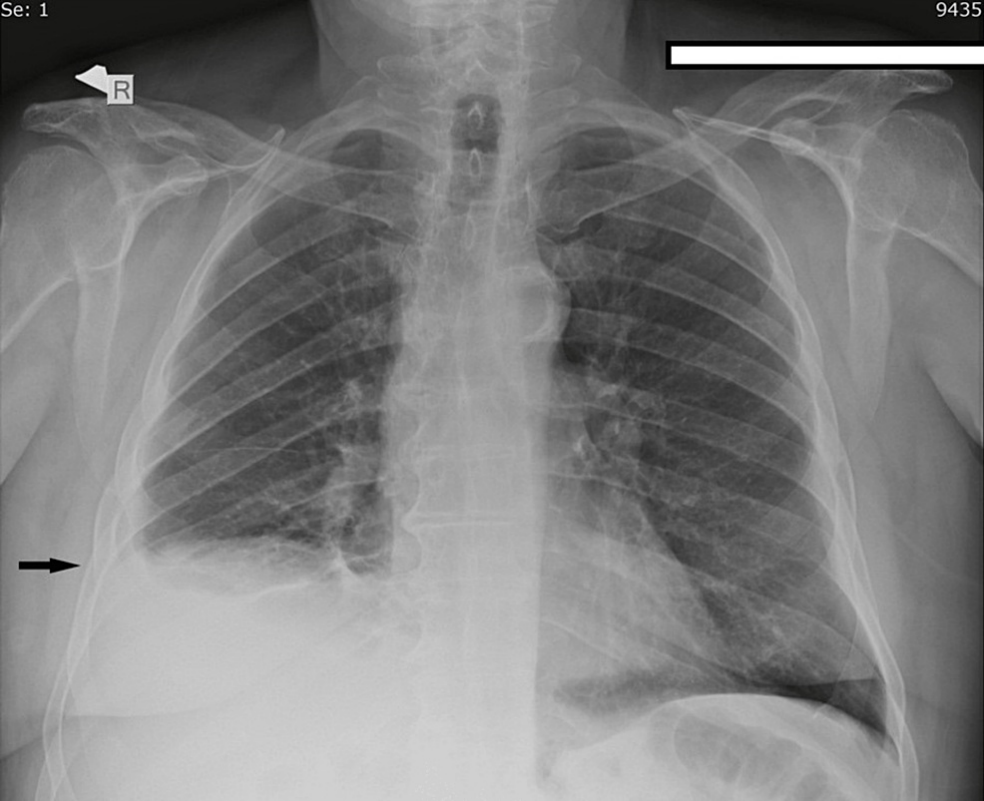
Chest X-ray on admission Elevation of right hemidiaphragm and blunting of right costophrenic angle.
